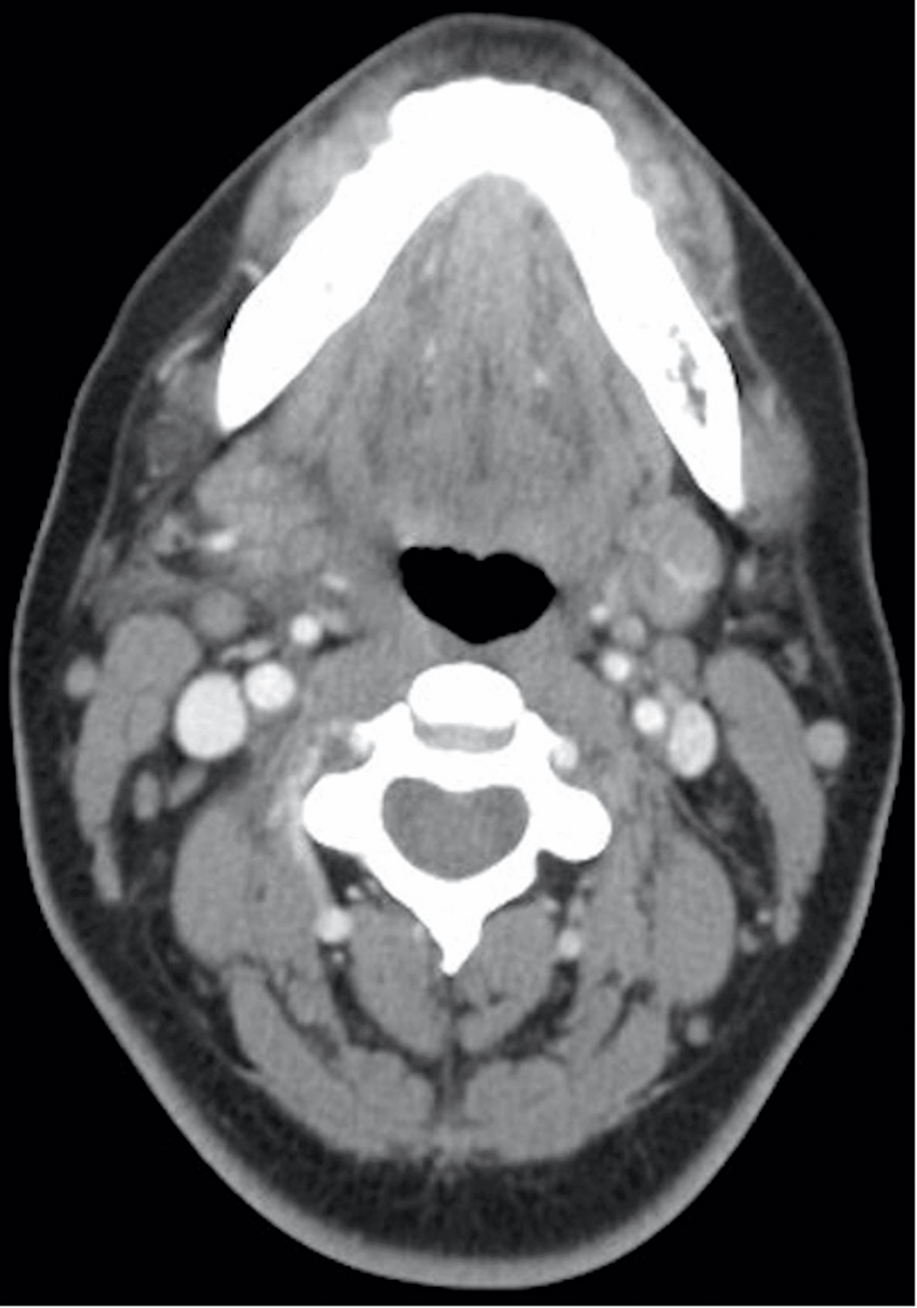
Computed tomography scan demonstrating submandibular sialadenitis.
